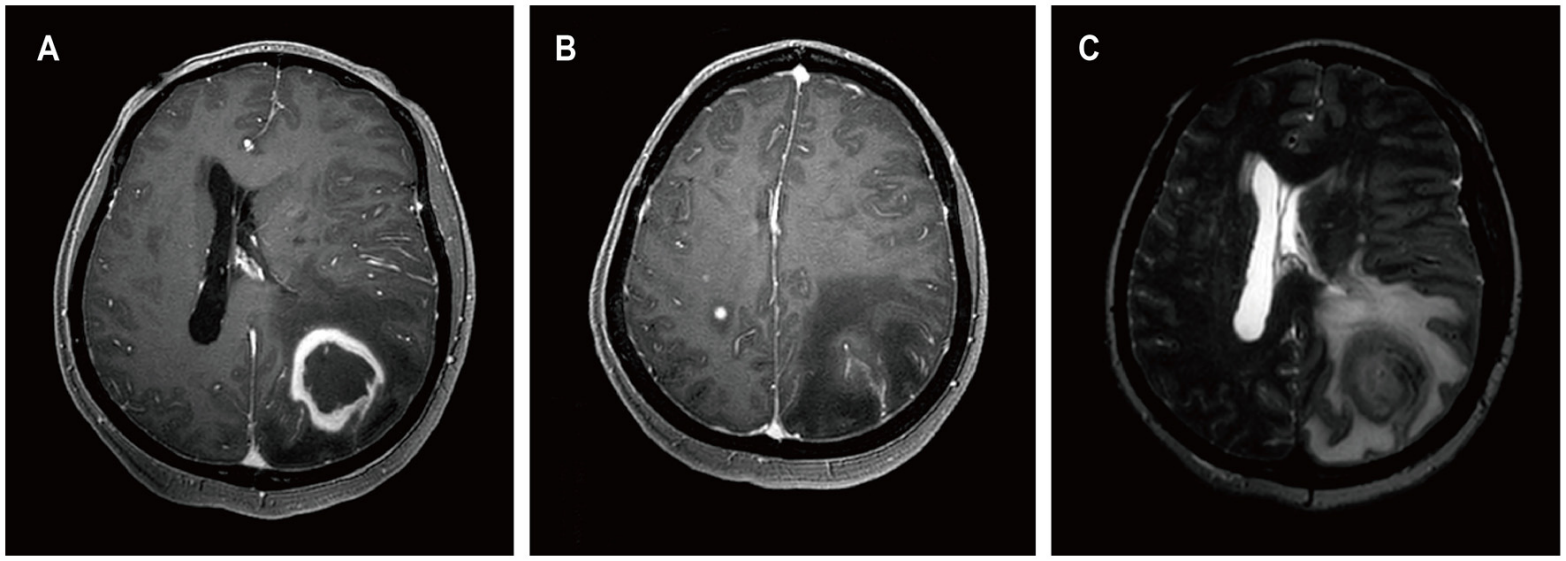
MRI findings of patient 5. Preoperative enhanced MRI images showed a ring-enhancing lesion in the left parietal lobe on T1-weighted images (A), a circular lesion in the right parietal lobe (B), and extensive edema surrounding the lesion in the left parietal lobe on T2-weighted images (C).
